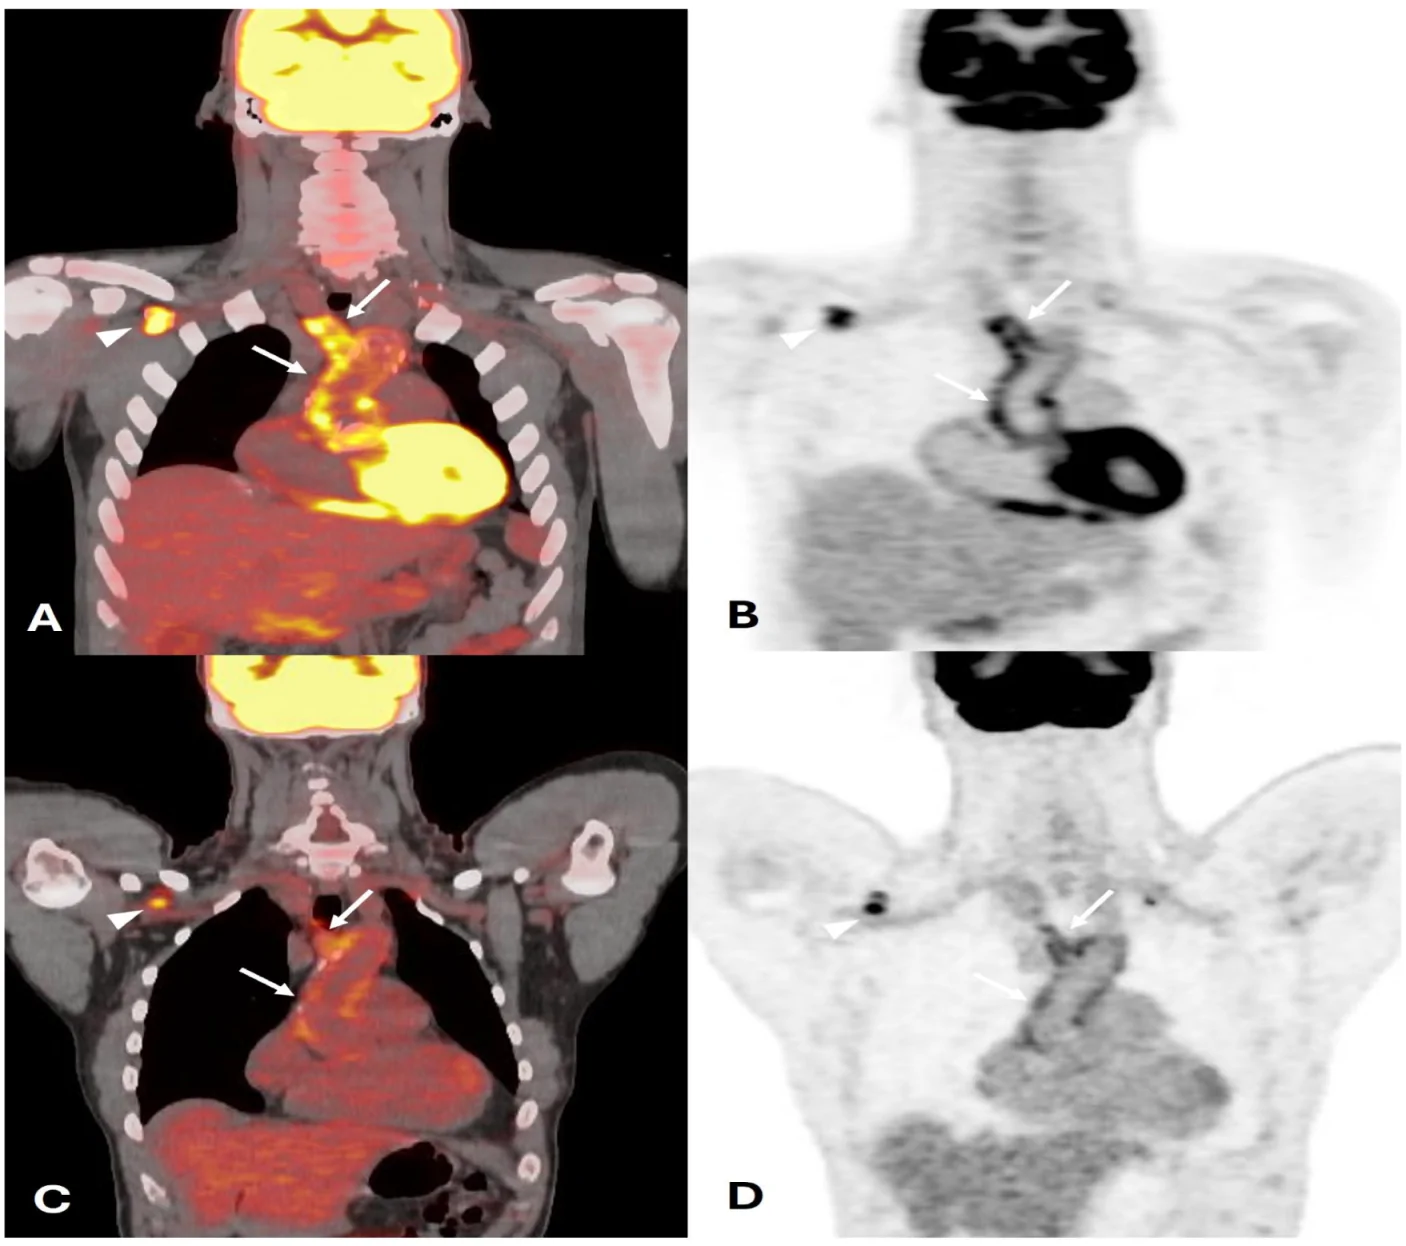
An example of Takayasu’s disease status post ascending aorta tubular grafting with recurrent vasculitis. Coronal fused and attenuation corrected PET images of F-18 FDG PET/CT studies at 2 different time points before and after immunosuppressive therapy with high-dose glucocorticoids. Pretherapy images (A,B) show intense abnormal radiotracer uptake throughout the aortic root, ascending aorta graft, brachiocephalic artery, and aortic arch (arrows) consistent with active vasculitis. Post-therapy images (C,D) show significantly improved radiotracer uptake involving the same vessels, consistent with decreased inflammation (arrows). In addition, focal tracer activity in the distal right subclavian artery (arrowheads) also improved after initiation of therapy.
